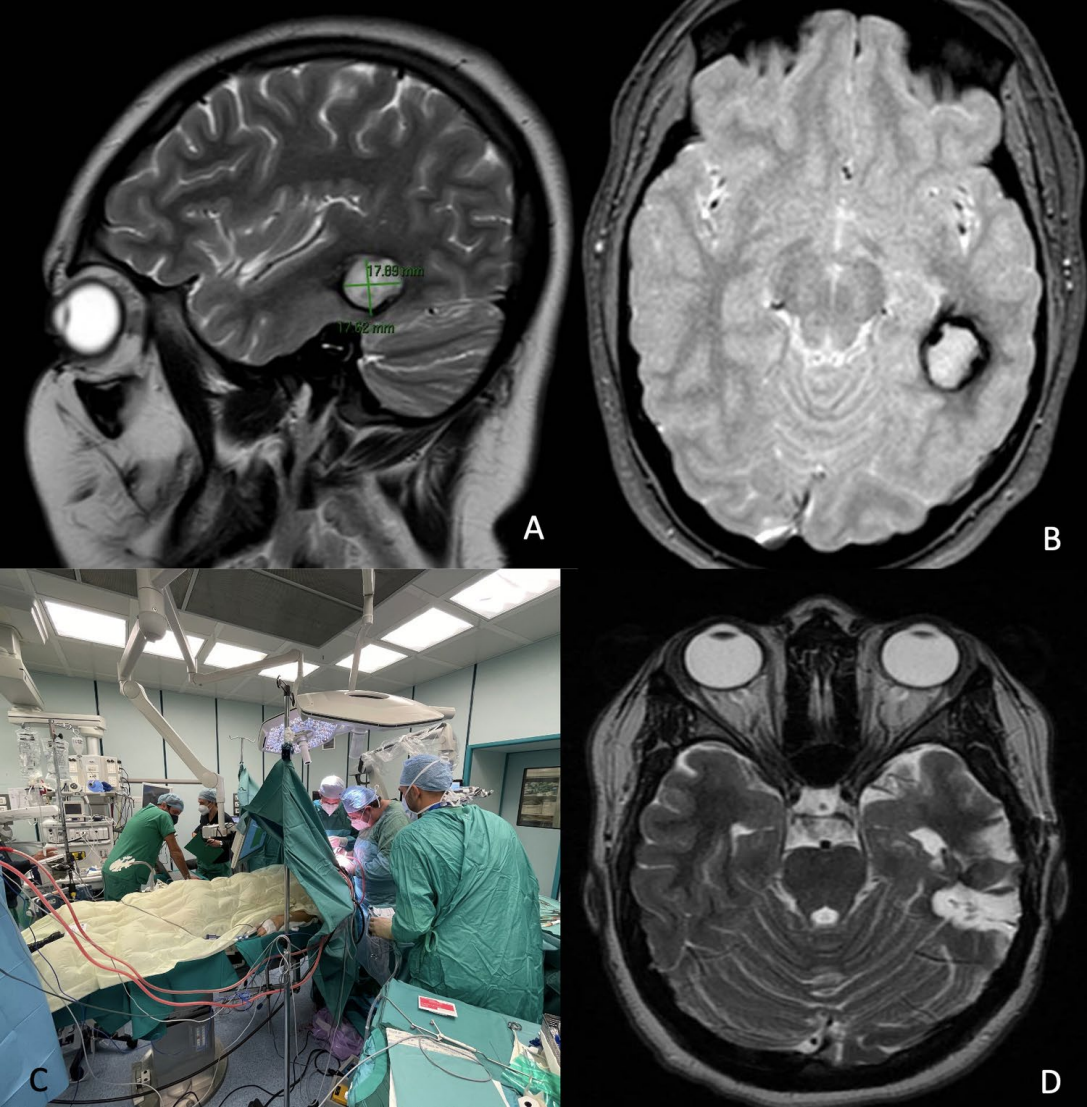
Cavernoma case number 3. A, B Pre-operative brain MRI showing the cavernoma in the left posterior temporal lobe with an adjacent developmental venous anomaly. C Intraoperative setting for awake surgery with collaboration of neurosurgeons, neurologists, and IONM team. D Post-operative MRI at 4 years showing complete excision of the malformation
